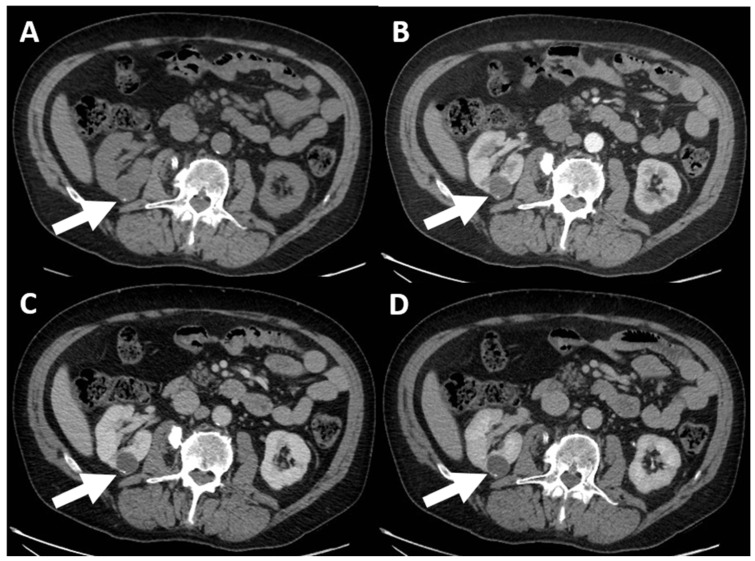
CT evaluation of small right renal cortical cystic lesion. The non-contrastographic (A), corticomedullary (B), nephrogenic (C), and excretory (D) phases show homogeneous cystic lesion with non-enhanced thin walls and calcification (arrows). The findings are consistent with Bosniak Class II.
